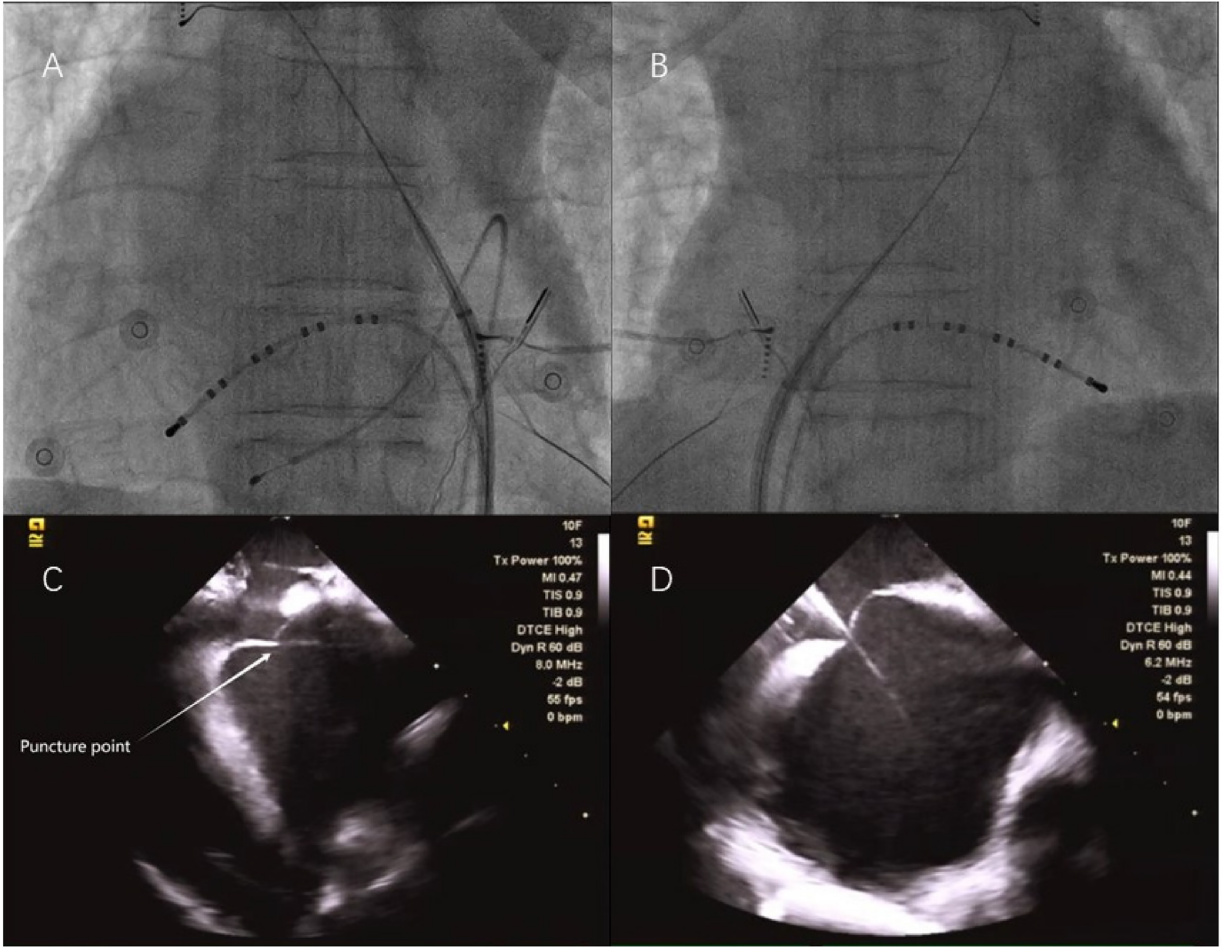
(A) X-ray image of the first transseptal (TS) puncture at position A–P; (B) X-ray image of the second TS puncture, taken at a 180° mirror image; (C) Intracardiac echocardiography (ICE) image of the first TS puncture; and (D) ICE image of the second TS puncture.
